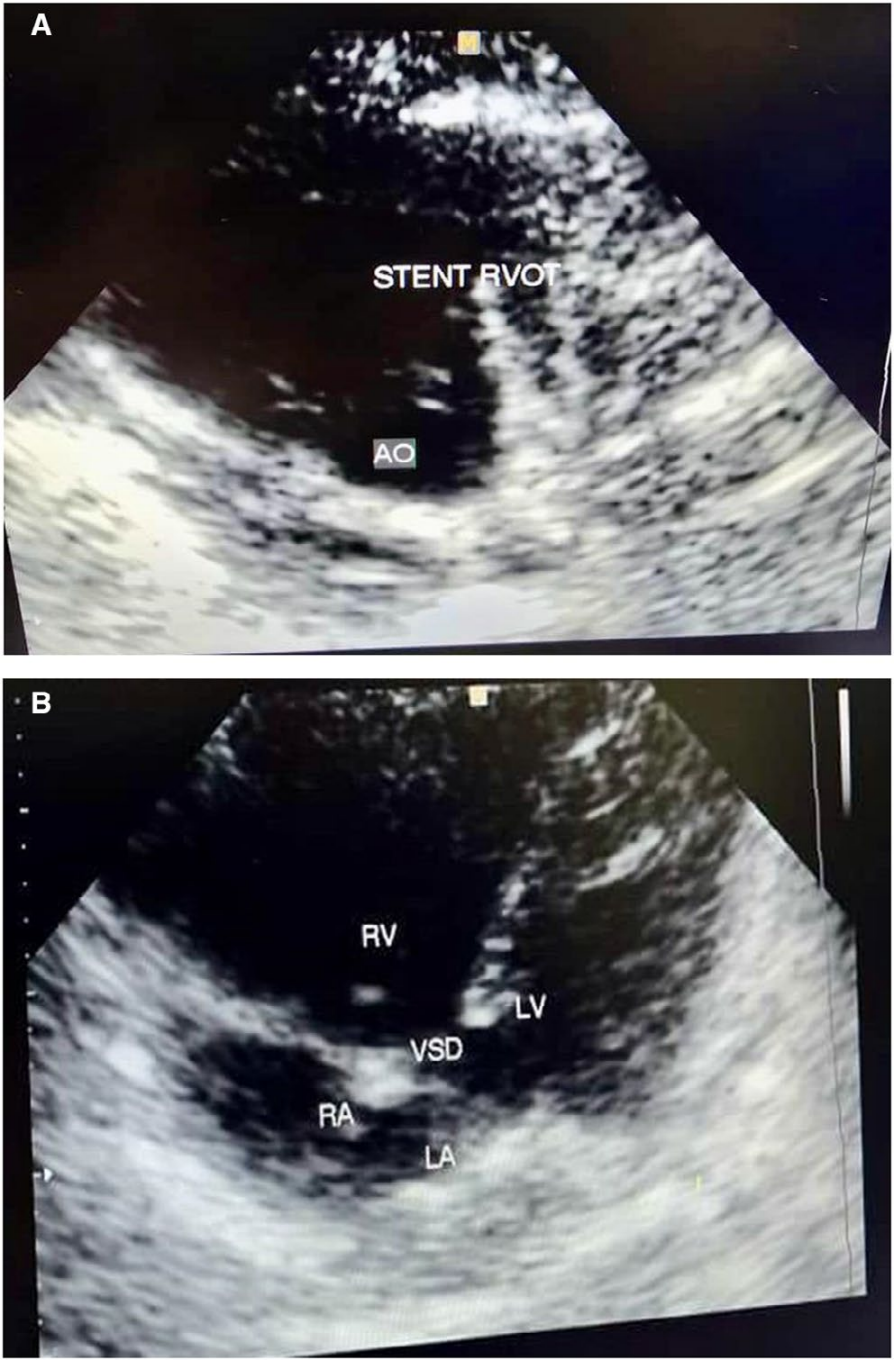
(A and B) Echocardiography after stent placement surgery.
